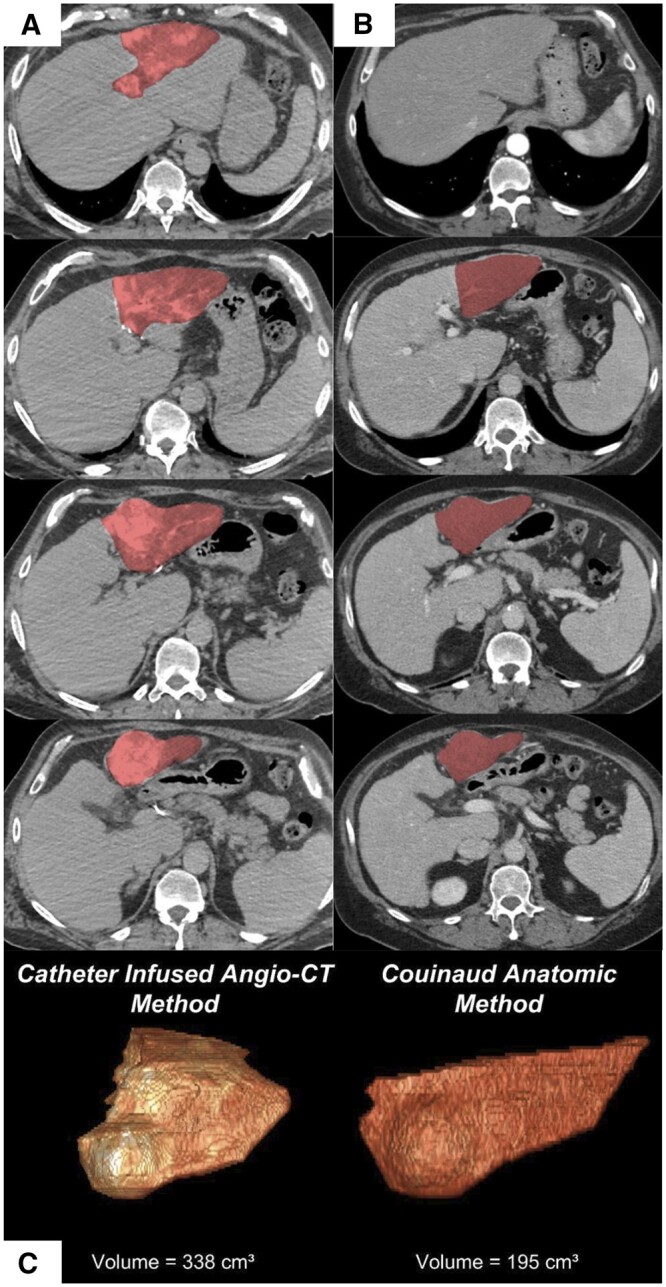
Demonstration of the differences between (A) volumetric measurement from angiosome acquired by Angio-CT vs (B) volumetric measurement derived from conventional Couinaud segment anatomy (portal venous phase; sample of contiguous cranial-to-caudal slices). (C) 3D reconstructions of the resultant delineated anatomy. In this case, the HCC tumour was visualized within segment 3; however, its tumoural angiosome is seen extending into segments 2 and 4 on Angio-CT. The unaccounted difference leads to underestimation by the Couinaud anatomic model (195 mL vs 338 mL). Note that the tumoural angiosome extends into segment 2 (red highlighted area on Angio-CT), whilst the corresponding area on diagnostic CT is not colored red for comparative purposes.
